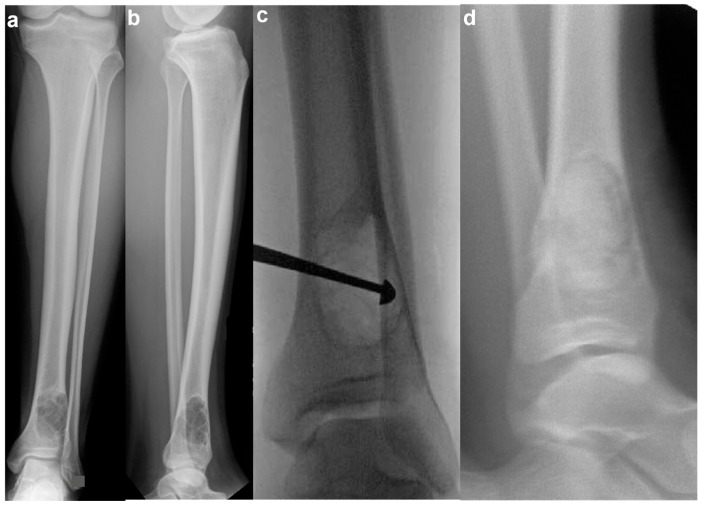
Surgical management of a symptomatic NOF. Pre-operative AP (a) and lateral (b) radiographs of a 17-year-old boy with a large, symptomatic left distal tibia NOF. Intra-operative fluoroscopy (c) demonstrating an AP radiograph with a curette in place. Post-operative lateral radiograph (d) demonstrating bone grafting in place.
